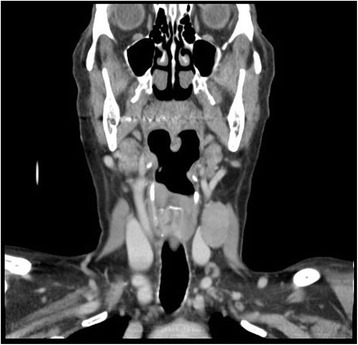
Computed tomography (coronal plane) of the patient’s left lateral neck mass
